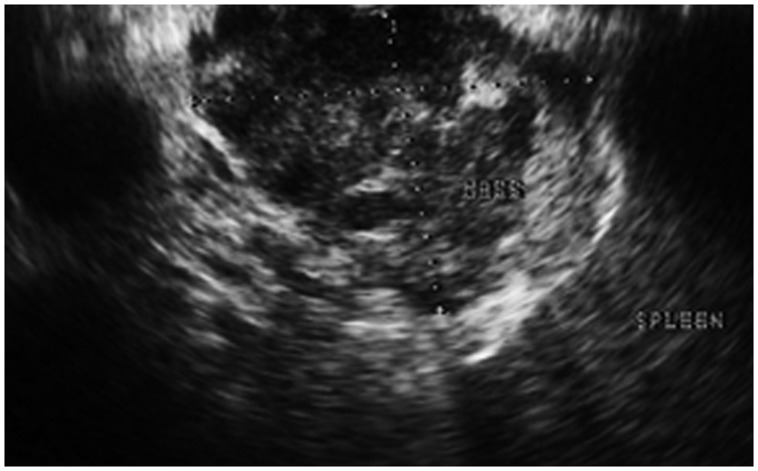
Endoscopic ultrasonography shows the pancreatic-tail mass, with mixed echogenicity and areas with cystic components suggestive of necrosis.
